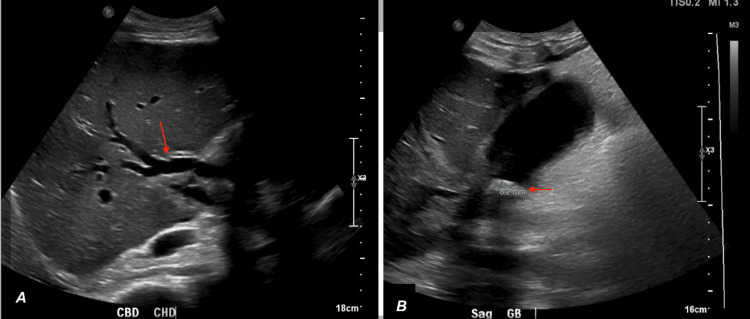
Abdominal ultrasound showing (A) intrahepatic biliary dilation and (B) gallstone at the neck of the gall bladder
